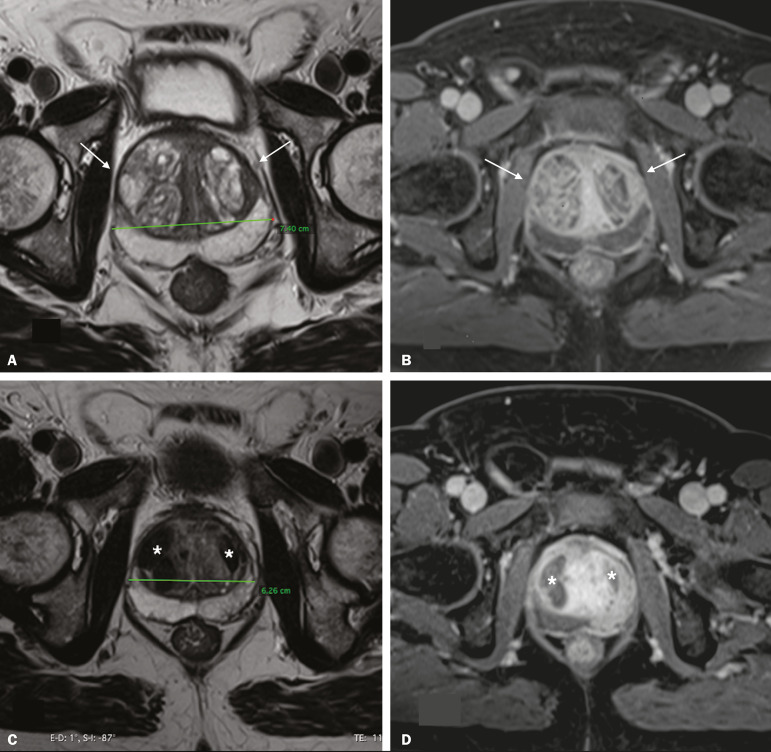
T2-weighted MRI sequence and contrast-enhanced T1-weighted MRI sequence with fat suppression (A and B, respectively), showing a markedly enlarged prostate due to bilateral BPH nodules in the transitional zone (arrows). C,D: T2-weighted MRI sequence and contrast-enhanced T1-weighted MRI sequence with fat suppression, both obtained after PAE (C and D, respectively), showing a reduction in prostate volume and infarction of the BPH nodules bilaterally (asterisks).
